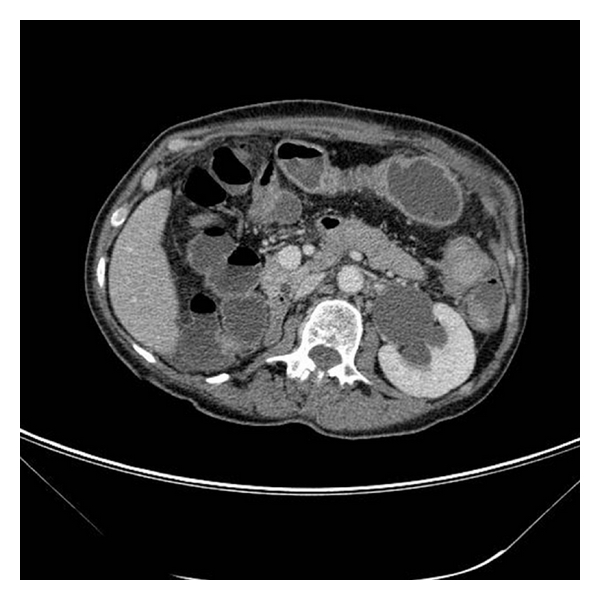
CT scan reveals left hydronephrosis secondary to compression on lower ureter by a rectal mass, previous right nephrectomy.
